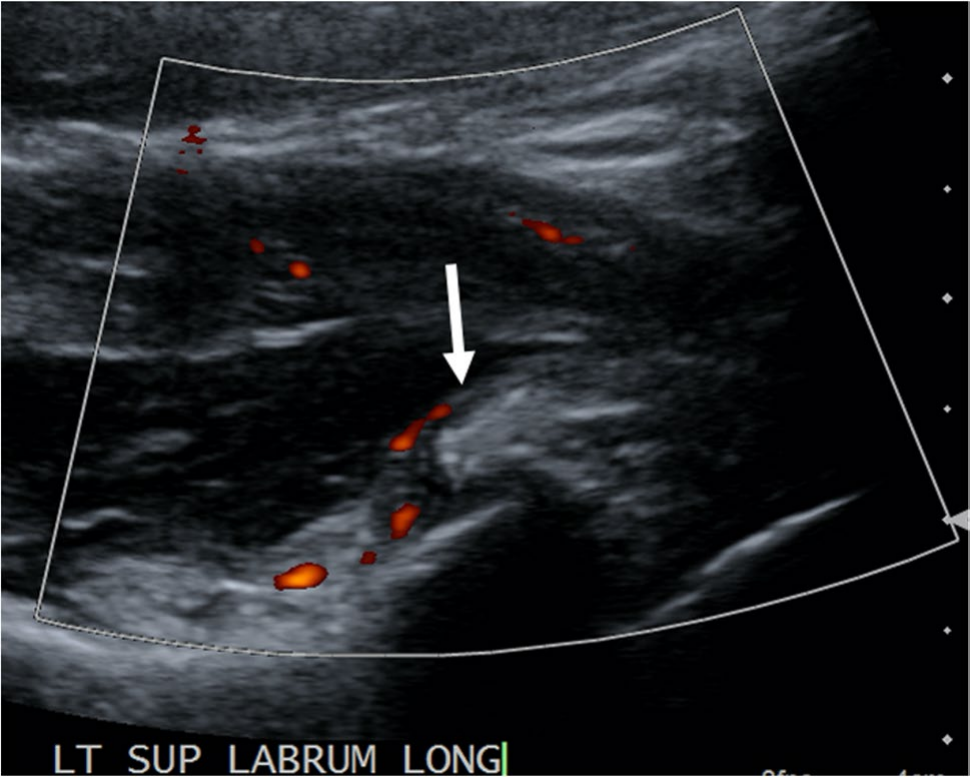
A 40-year-old male presented with a diagnosis of ACP of the left shoulder based on the findings of an MRI for ultrasound-guided corticosteroid injection. A longitudinally orientated image using color Doppler was obtained prior to the injection which demonstrates a focus of capsular calcification adjacent to the anterosuperior glenoid rim and associated capsular/pericapsular hyperemia
